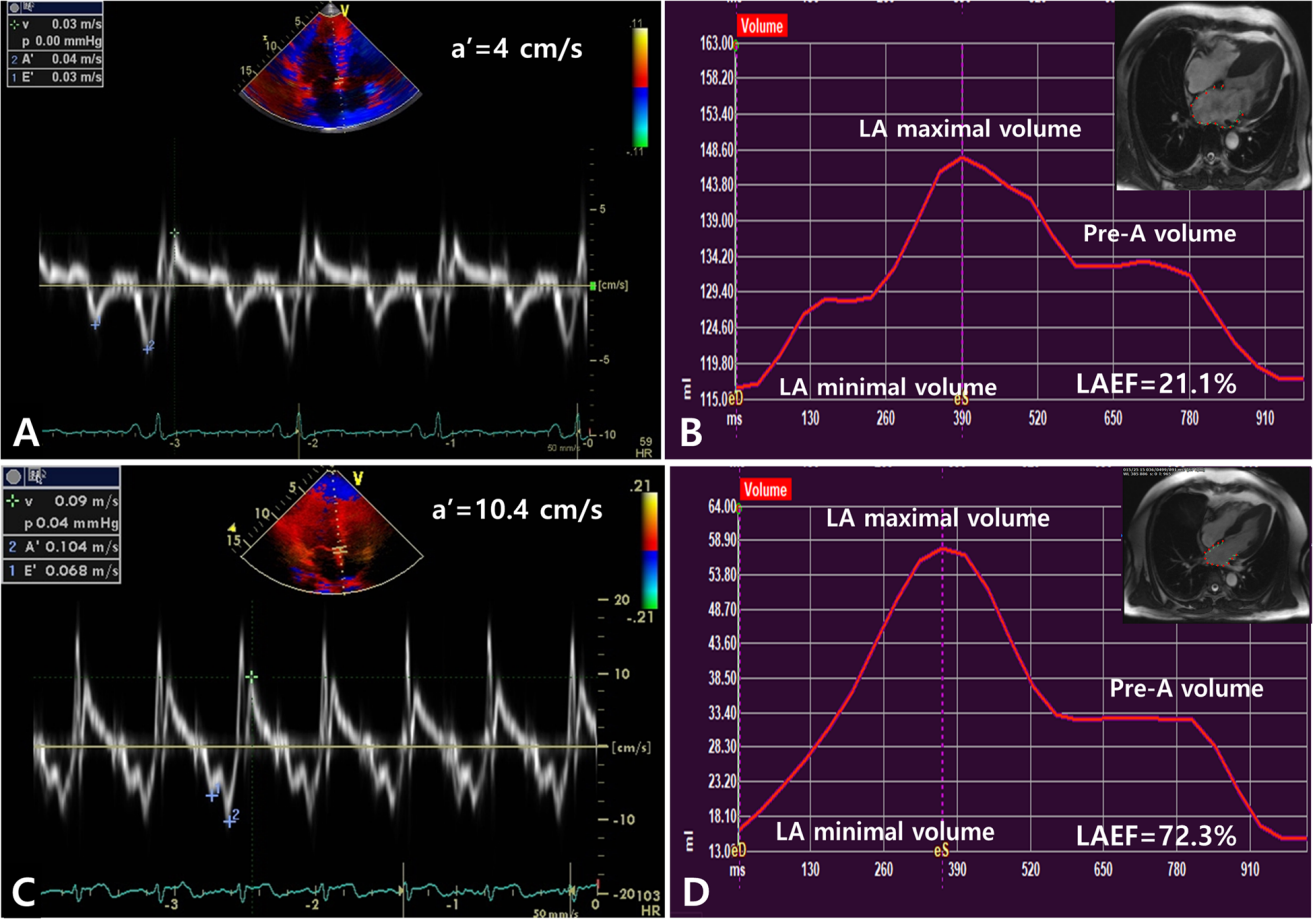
Tissue Doppler index (right panel) and phasic left atrial function measurement with cardiac magnetic resonance imaging (right panel) in a patient with pathogenic sarcomere gene mutation (*TNNI3* mutation, a, b) and without sarcomere mutations (c, d)
